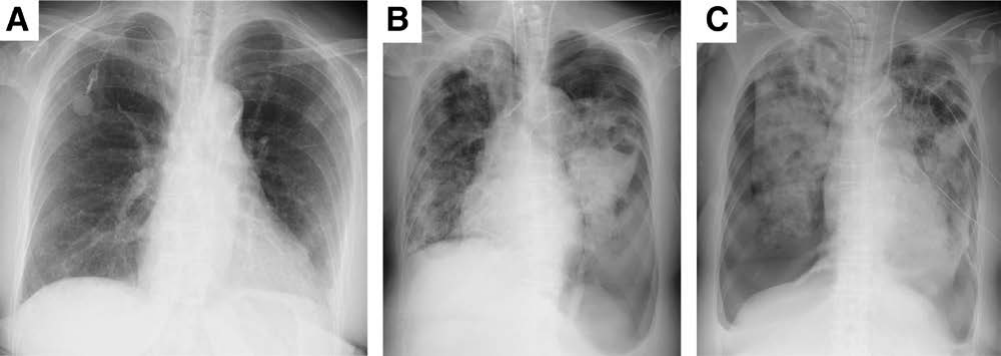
Chest X-ray time course of the case. (A) Recorded before this admission. The CV port was implanted in the right subclavian vein. No obvious lesions were found in the lung field. (B) Recorded on day 9. Left pneumothorax was found. (C) Recorded on day 19. Right pneumothorax was found in addition to existing left pneumothorax. Left pneumothorax persisted even with the insertion of 2 chest tubes. CV = central venous.
